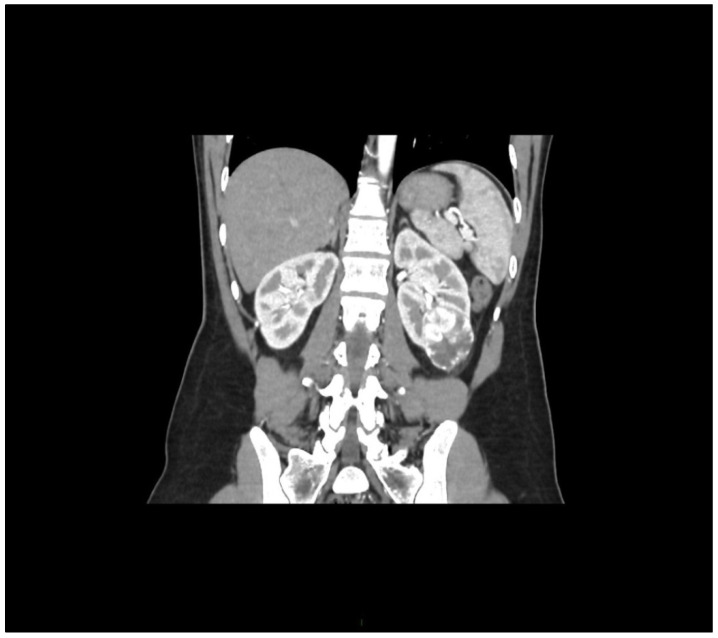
Arterial-phase multislice CT in the coronal plane shows a well-vascularized tumor at the lower pole of the left kidney.
